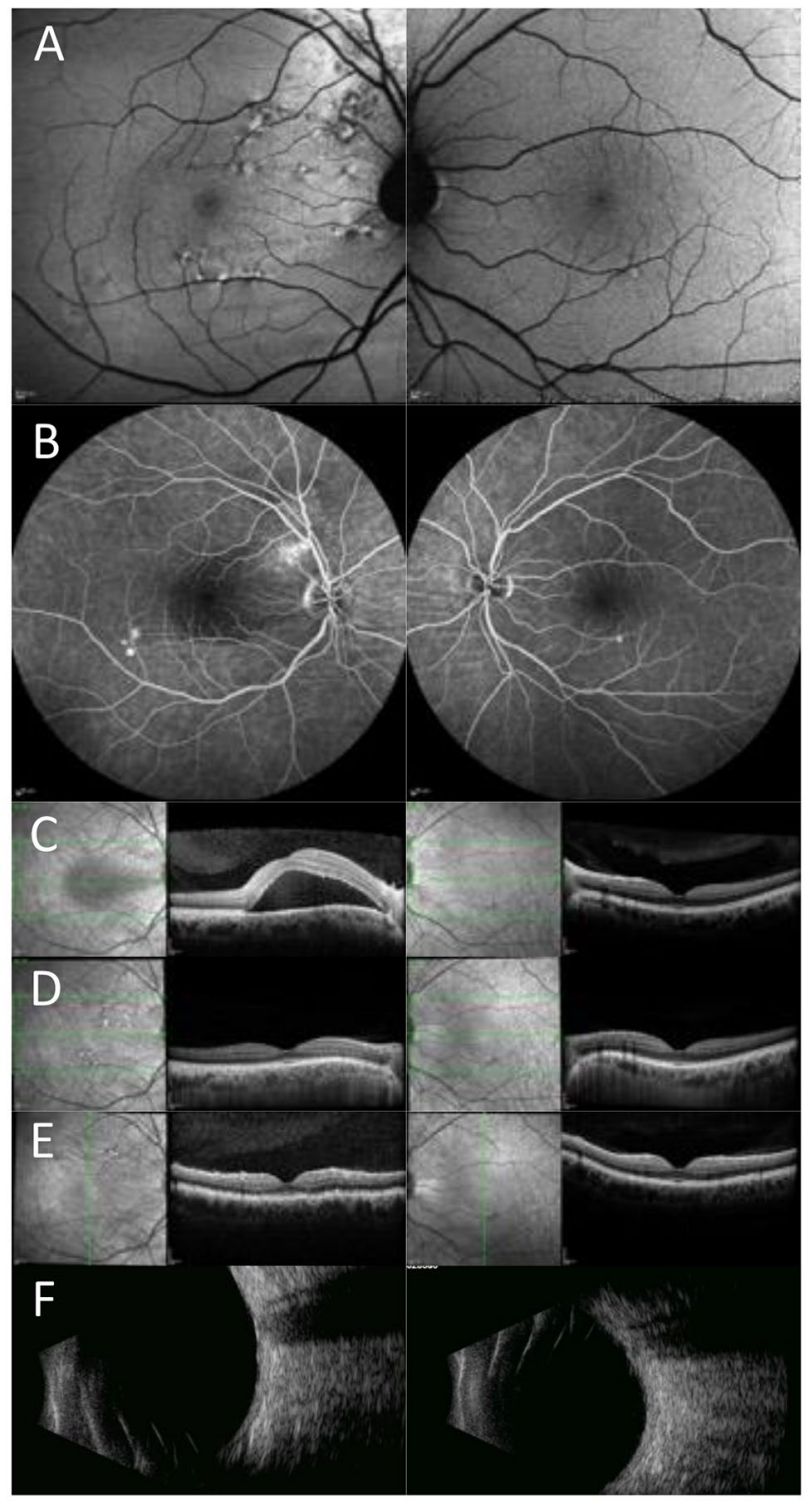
Multi-modal imaging for case 4 A) Fundus autofluorescence (FAF) imaging showing features of active focal CSC in the right eye and features of a previous CSC in both eyes (B) FFA imaging demonstrating CRF in macular region of the right eye and areas of hyperfluorescence consistent with chronic CSC. The left eye has CRF nasal to the optic disc and a hyperfluorescent spot consistent with CSC. (C-E) OCT imaging at baseline (C) shows typical neurosensory elevation of CSC in the right eye and follow up OCT imaging (D) shows resolution of the CSC in the right eye (E) OCT imaging with vertical scan shows the CRF in the macula of the right eye. (F) Ultrasound B scan images showing bilateral flattened posterior poles
